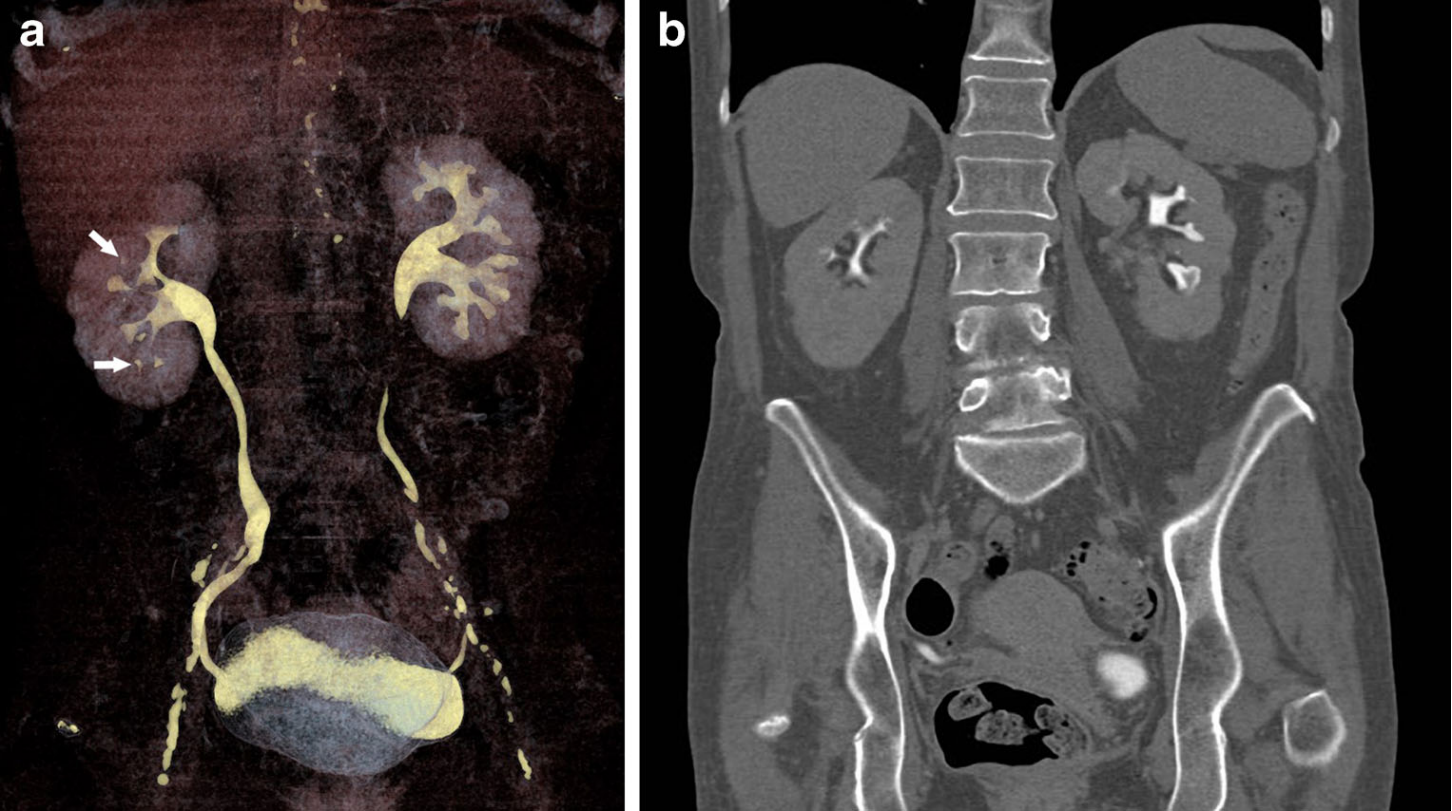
Sixty-two-year-old woman with node positive ductal carcinoma undergoing treatment with carboplatin and paclitaxel. (a) Coronal maximum intensity projection (MIP) image showing bilateral blunting of the calices consistent with renal papillary necrosis. (b) CT-urographic image demonstrating bilateral irregularly shaped, projections at the apex of the left renal pyramids suggestive of papillary necrosis
